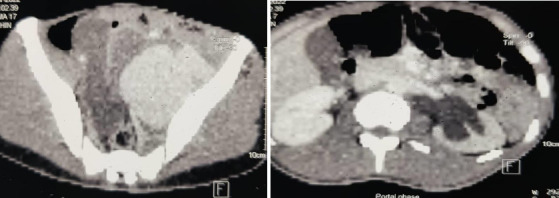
Pre-operative CT angiogram of patient 3
